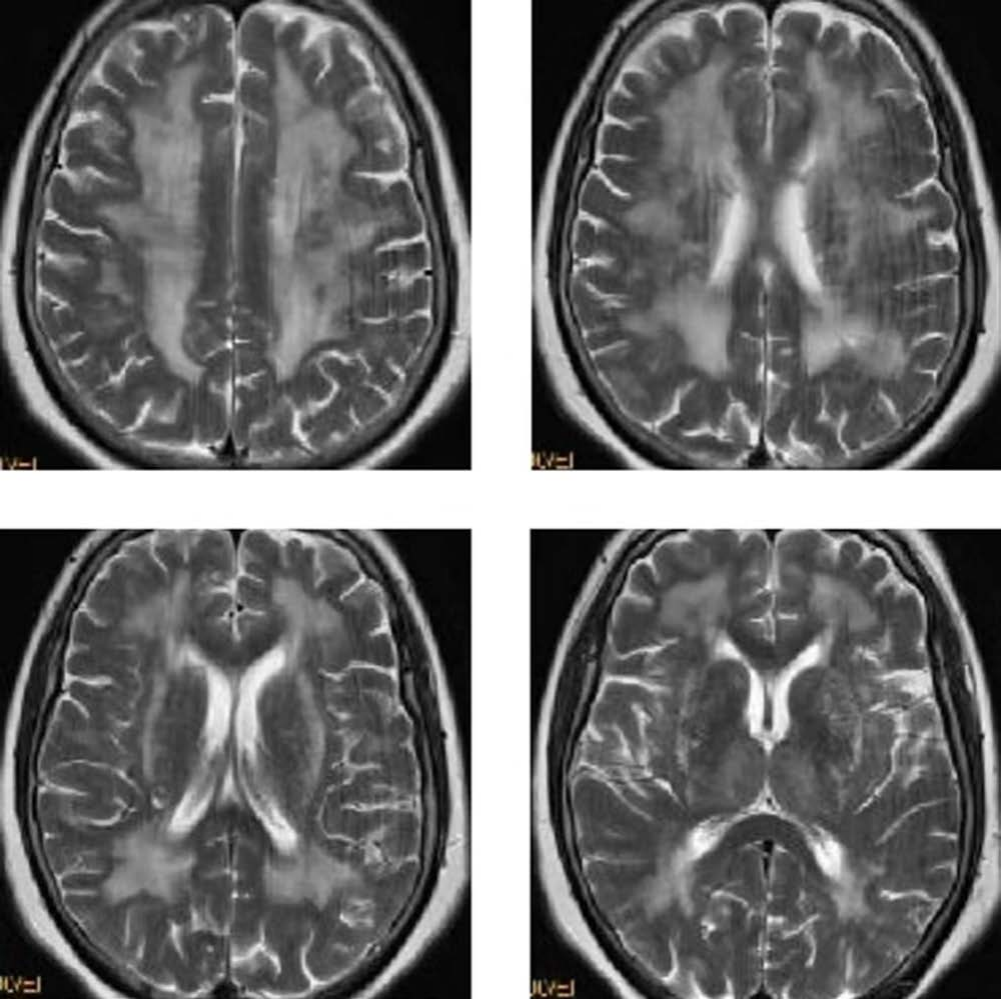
Cerebral MRI image of the patient’s mother’s brain. MRI = magnetic resonance imaging.
